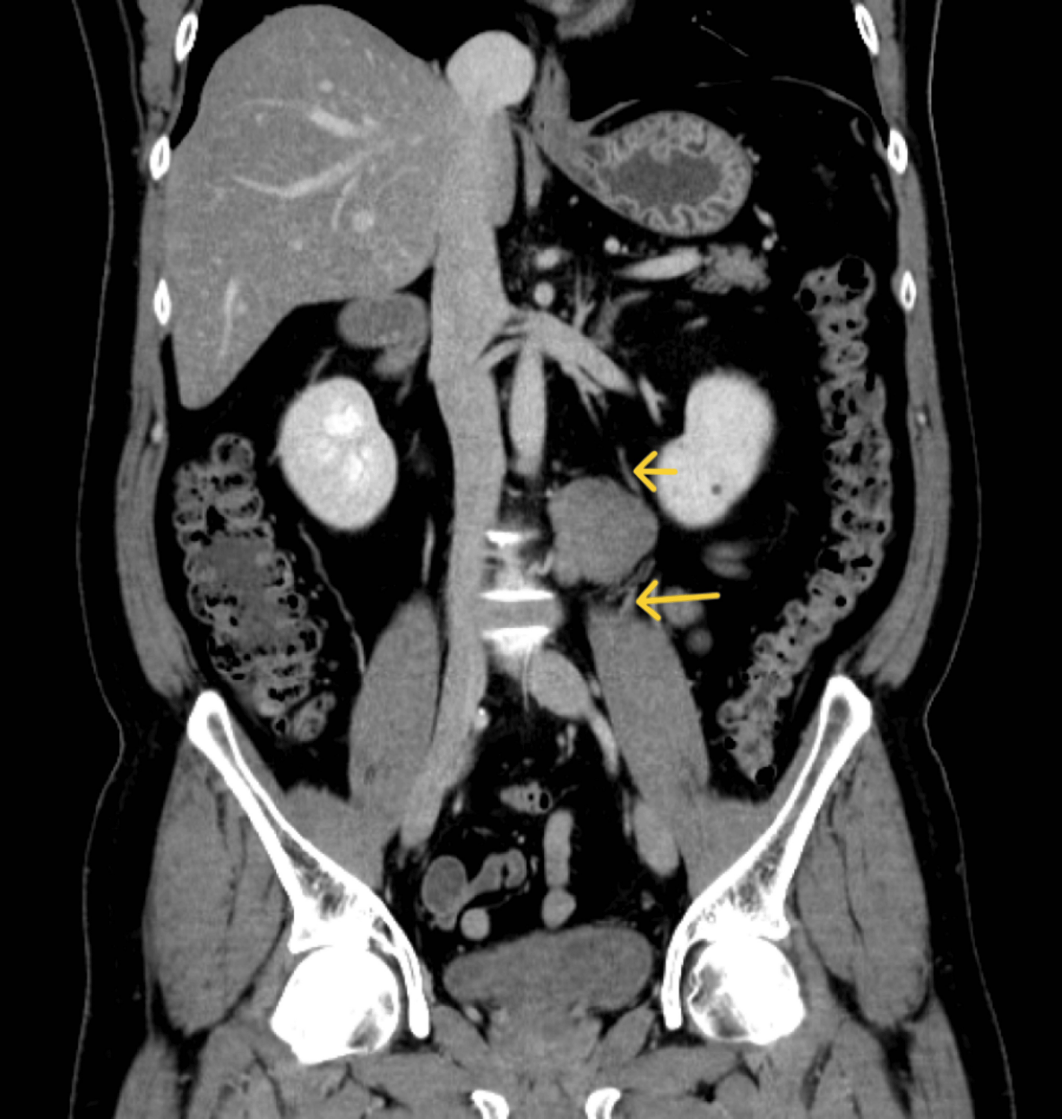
Preoperative contrast CT scan The tumor is in contact with the left ureter (arrows) CT: computed tomography
